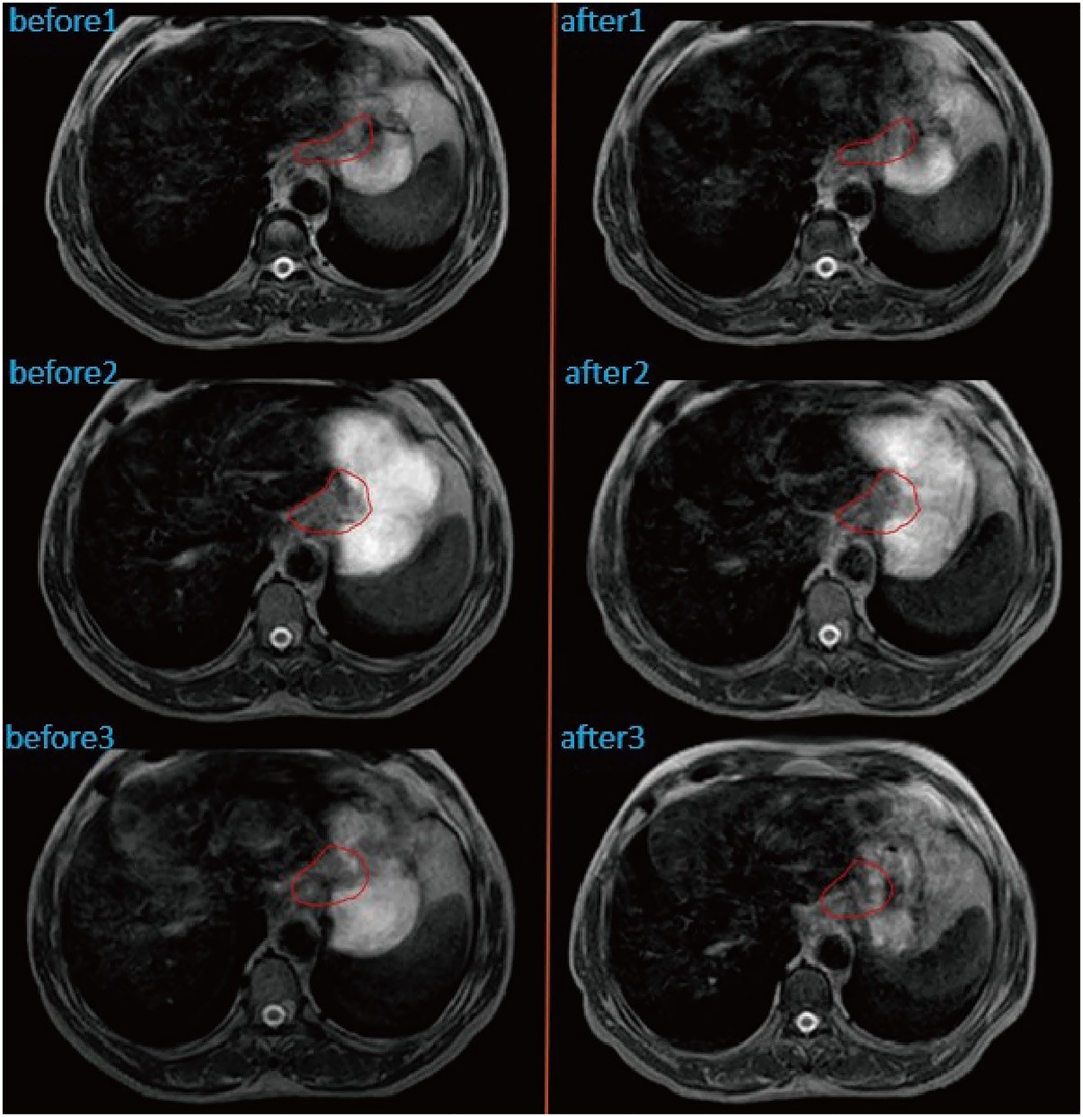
Intra-fractional changes of the anatomical structures by performing MRI before and after treatment at three different fractions. GTV was outlined in red.
